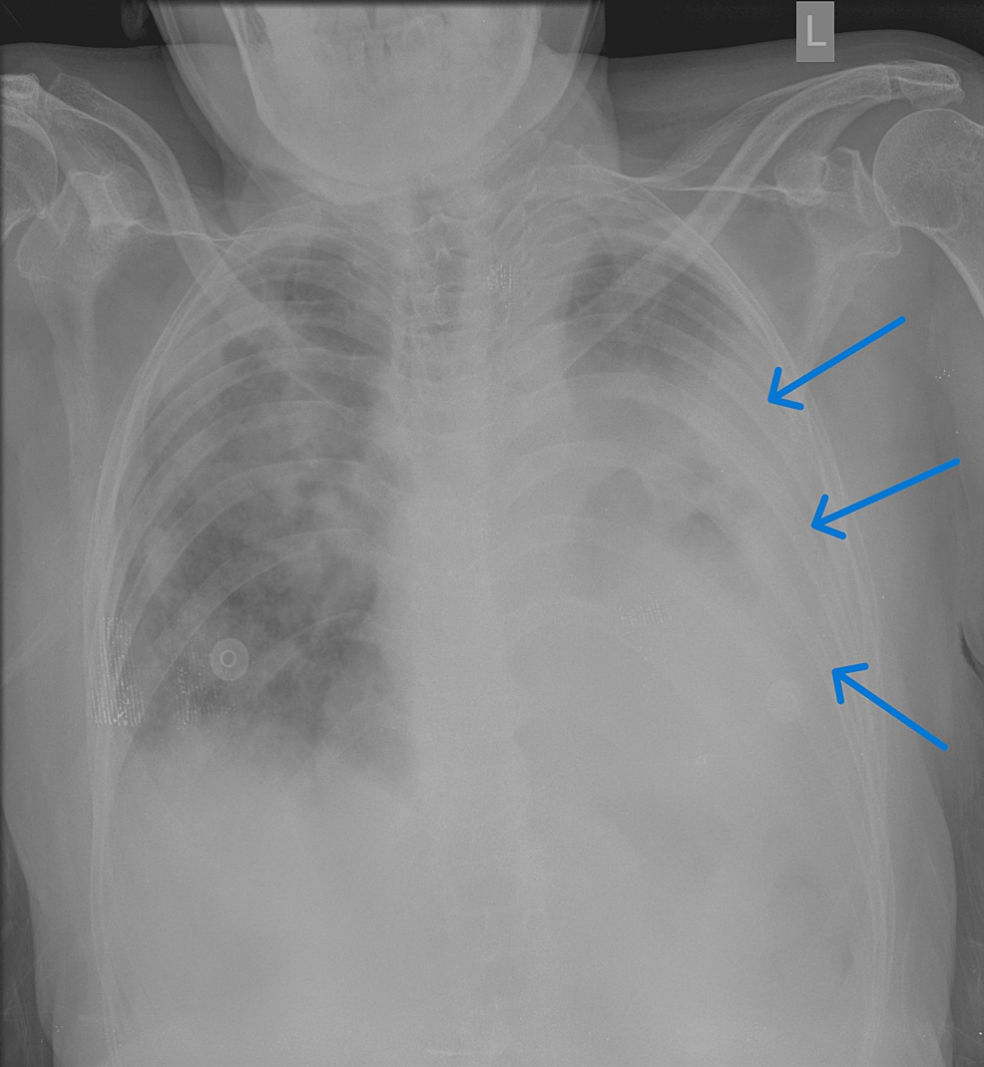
Chest x-ray during the subsequent admission showing an increase in the opacity on the left hemithorax (blue arrows)
